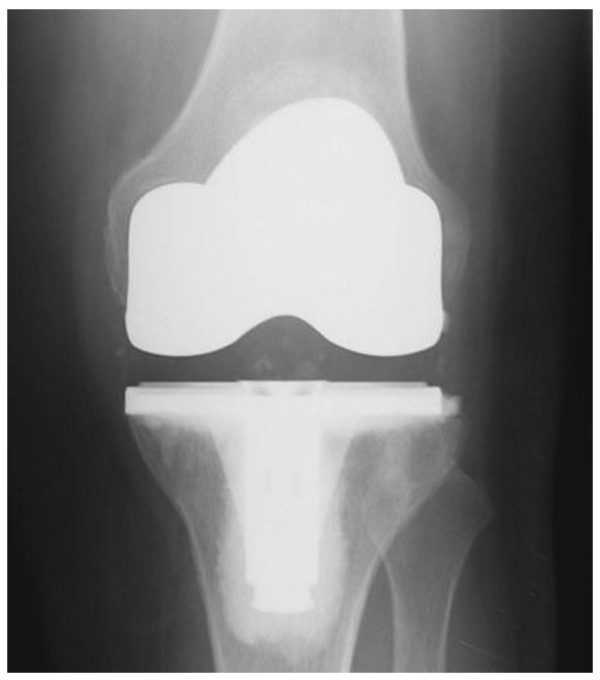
AP radiograph showing a Total Knee Arthroplasty.
